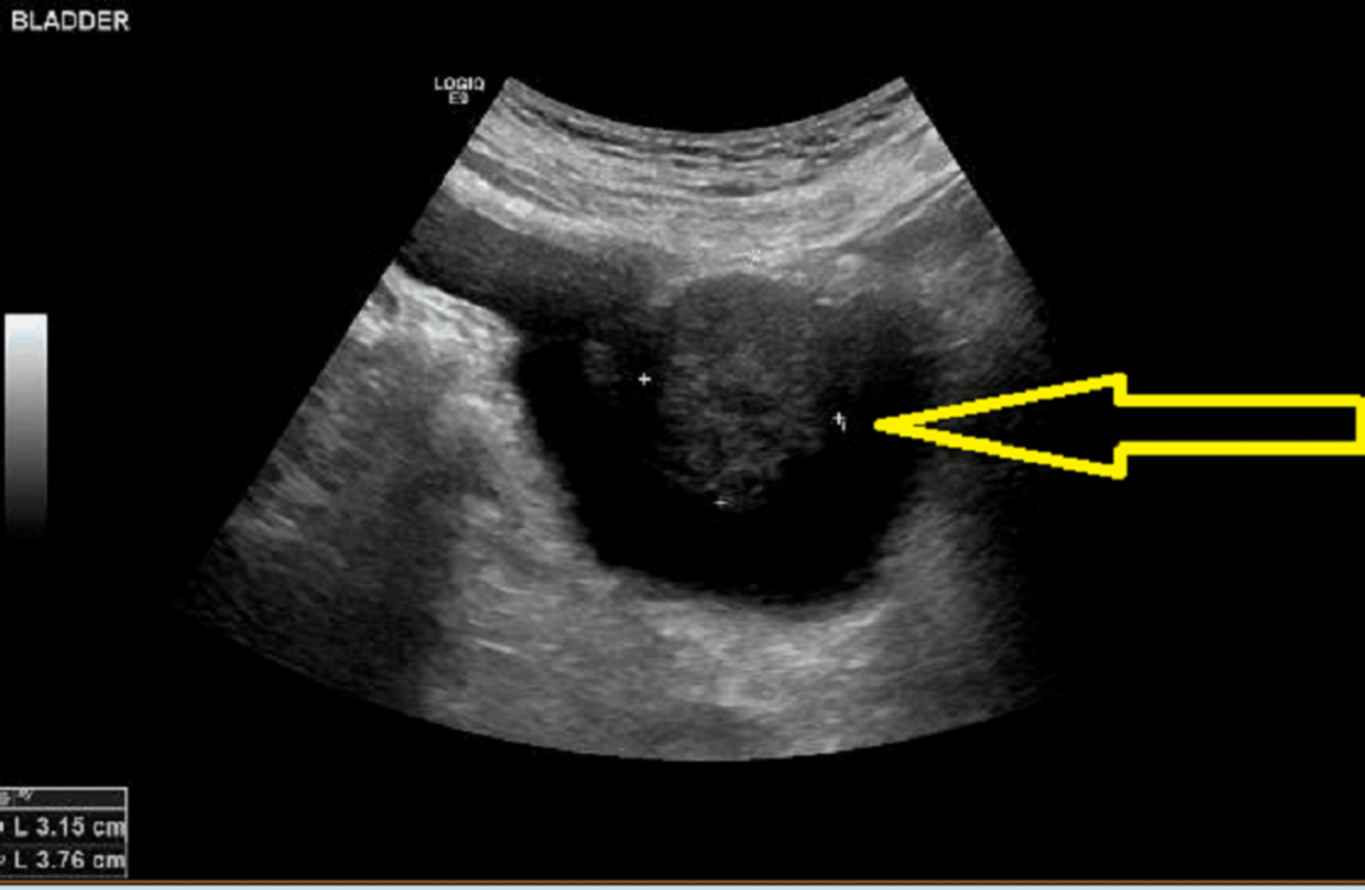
Ultrasound scan of the bladder showing a hypoechoic lesion in the bladder
